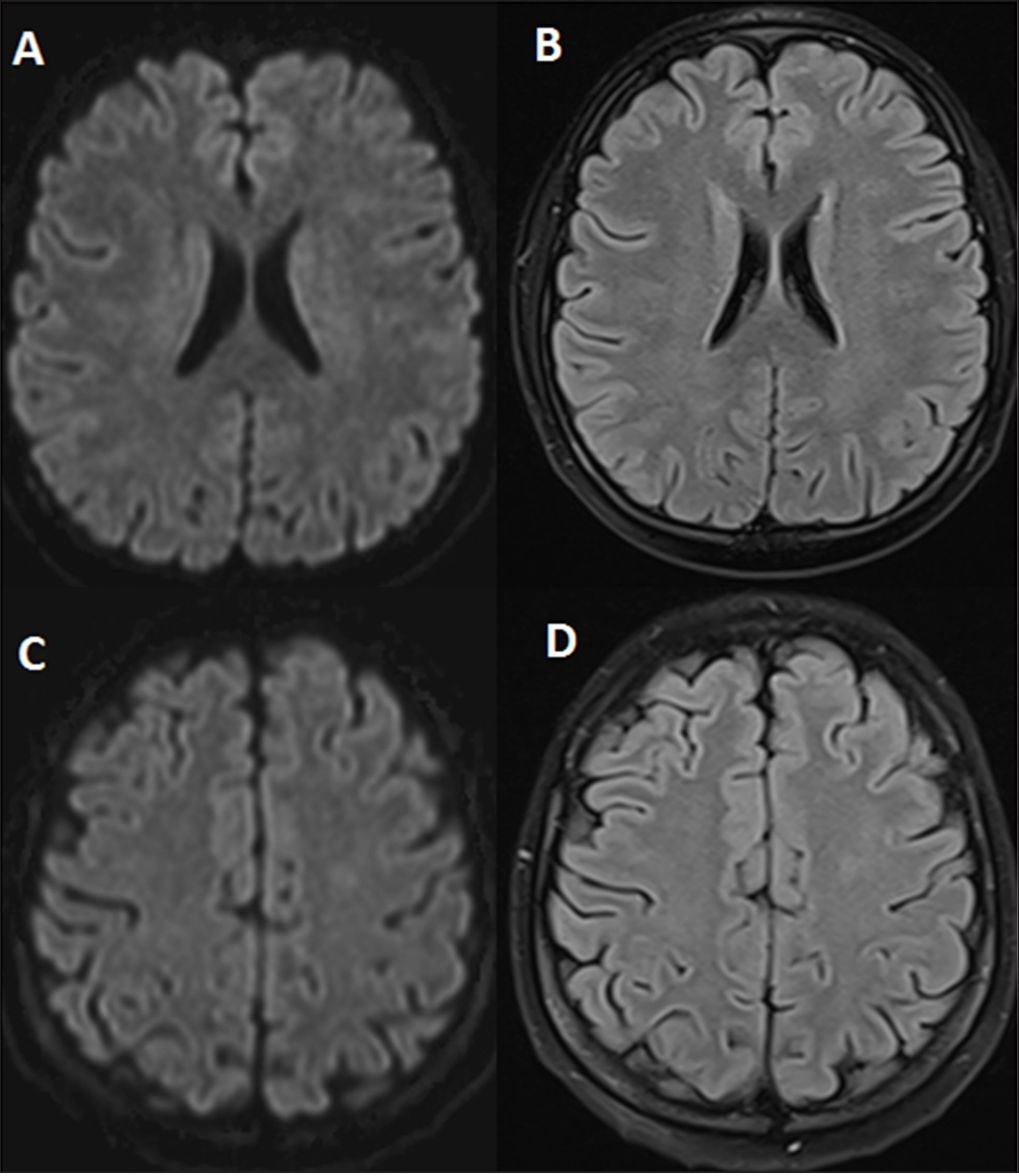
Follow-up brain MRI at four weeks showing complete resolution of radiological abnormalities (A, C: axial diffusion-weighted imaging; B, D: Axial fluid attenuated inversion recovery images).
